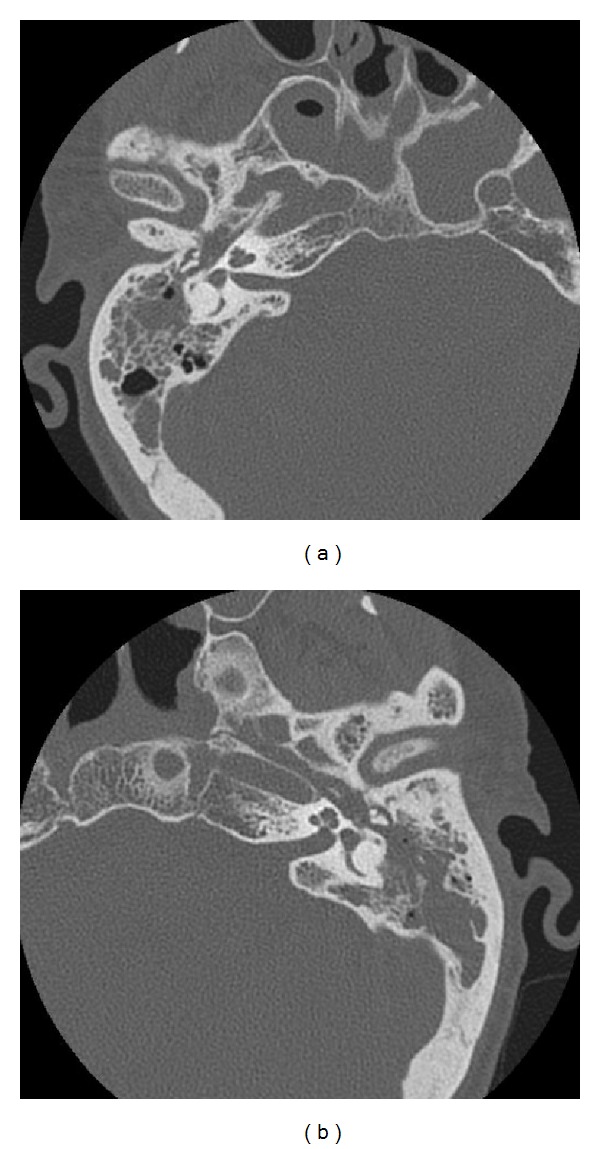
CT scan showing inflammatory tissue filling both left and right tympanic cavities.
